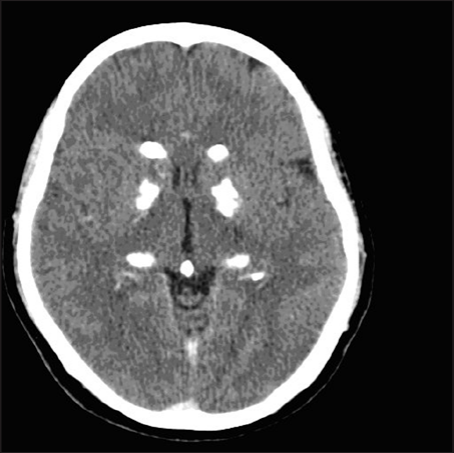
CT scan of the brain: Transverse view shows bilateral calcifications of the thalamus and corpus striatum.
